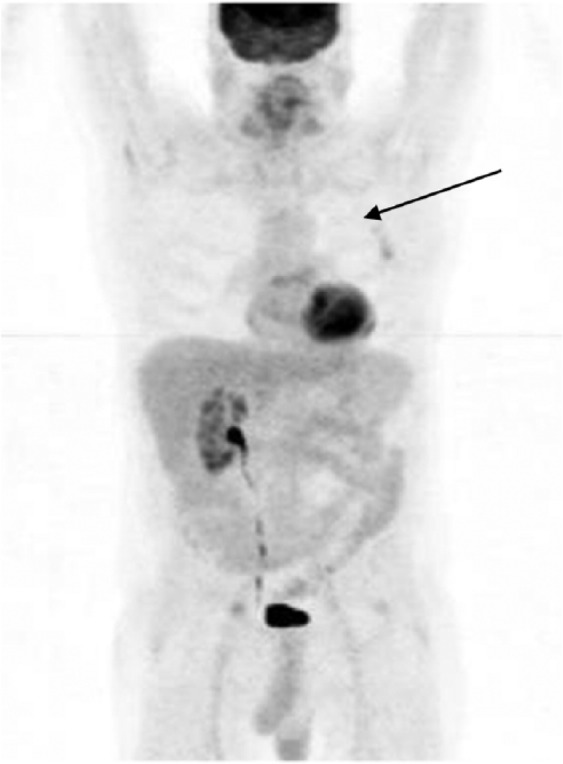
A repeat PET/CT scan in March 2012 showed complete resolution of the upper
